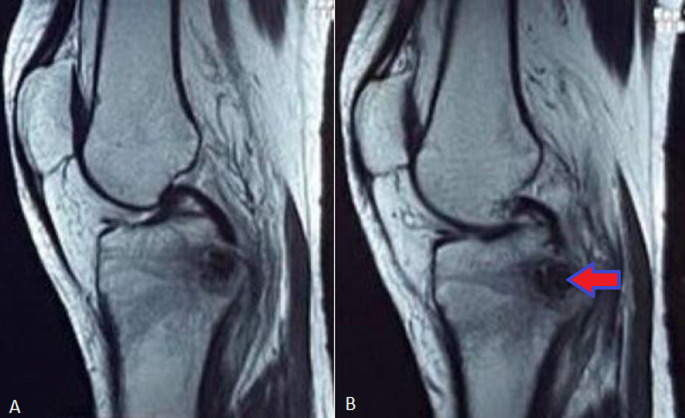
magnetic resonance imaging of right knee joint: A) presence of significant joint effusion in patella-femoral and tibia-femoral joint space; B) a focal, well defined altered signal intensity seen in the upper end of tibia with surrounded marrow oedema
